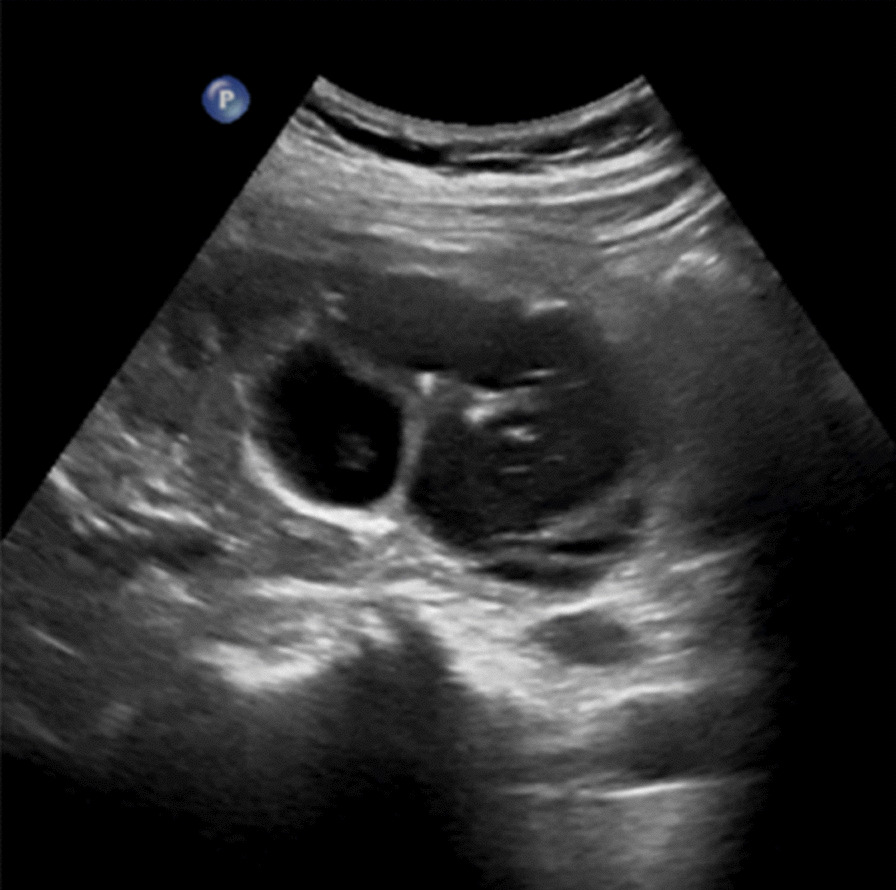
Ultrasonography demonstrating an cystic mass with thickened walls and irregular septa on the middle and the lower pole of the right kidney
